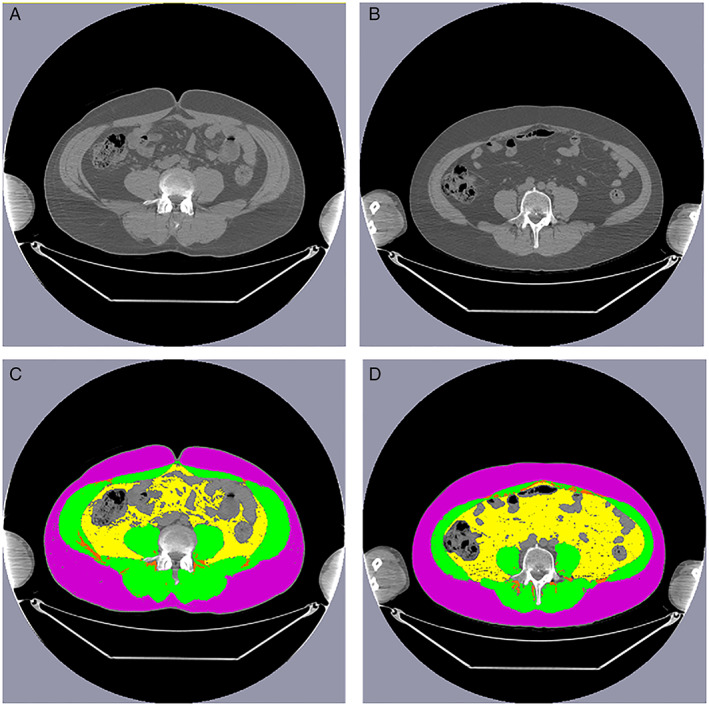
Axial computed tomography images of the third lumbar vertebra region without (top) and with (bottom) annotation showing skeletal muscle (green), subcutaneous adipose tissue (purple), visceral adipose tissue (yellow), intra‐muscular and inter‐muscular adipose tissue (orange) for patients (A) without and (B) with sarcopenic obesity. Characteristics of both patients: male, 50 years old at haematopoietic cell transplant, diagnosis: non‐Hodgkin lymphoma, BEAM conditioning, low (<3) haematopoietic cell transplant‐comorbidity index. Patient on the left (A): body mass index 29.3 kg/m^2^, skeletal muscle index 63.9 cm/m^2^, subcutaneous adipose tissue area 271.2 cm^2^, visceral adipose tissue area 53.0 cm^2^, intra‐muscular and inter‐muscular adipose tissue area 9.2 cm^2^; Patient on the right (B): body mass index 27.5 kg/m^2^, skeletal muscle index 46.7 cm/m^2^, subcutaneous adipose tissue area 267.9 cm^2^, visceral adipose tissue area 185.7 cm^2^, intra‐muscular and inter‐muscular adipose tissue area 7.8 cm^2^.
